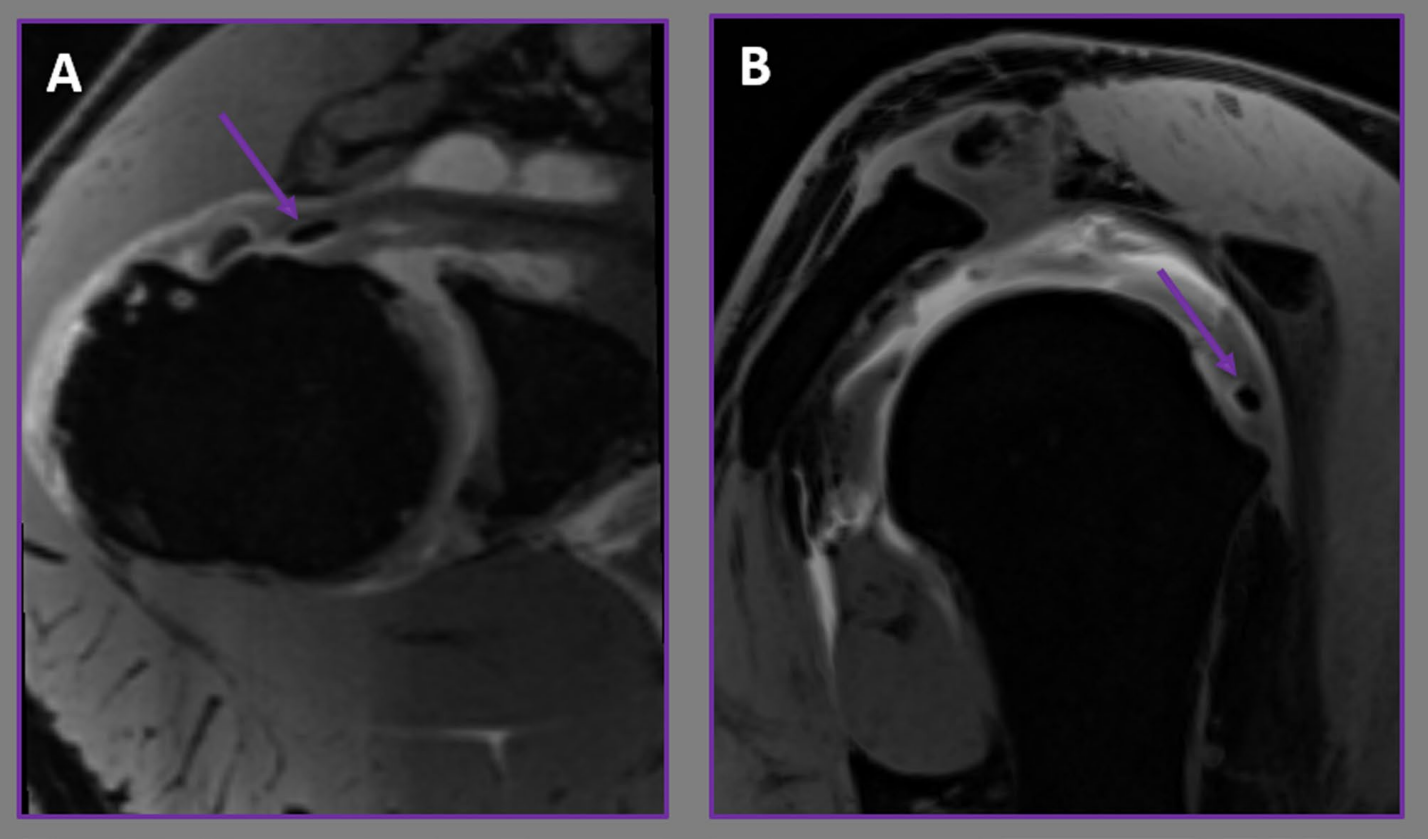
Axial (A) and oblique sagittal (B) fat-suppressed T1-weighted VIBE MR arthrograms show lesser tuberosity avulsion fracture (arrows) and its associated rotator cuff tendon rupture
